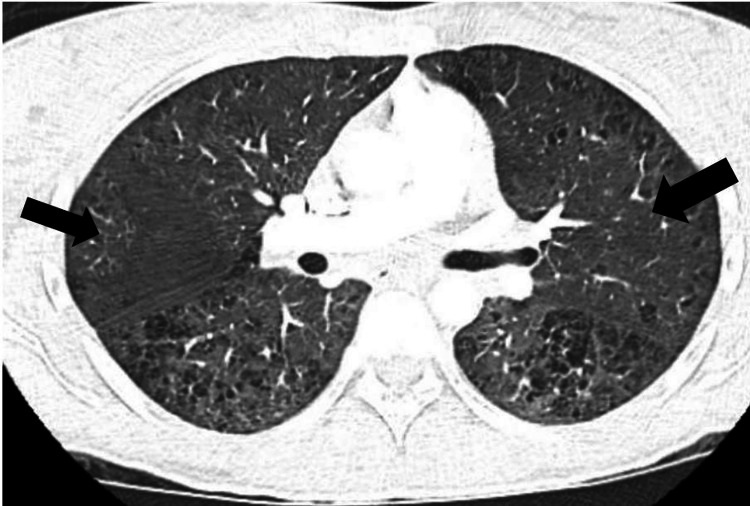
Typical appearance of cardiogenic pulmonary edema in bilateral lungs on CT chest
